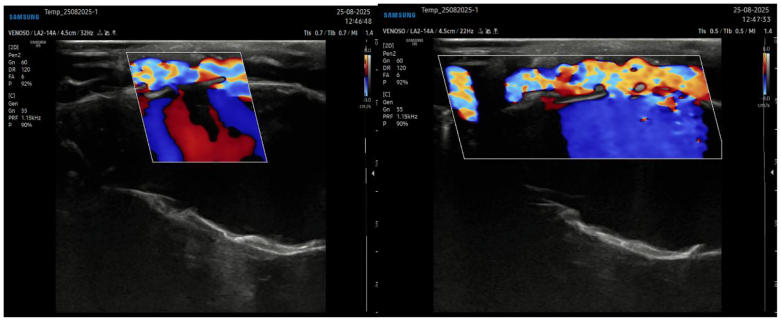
Intraoperative duplex ultrasound (DUS) examination. In the region of interest (ROI), the presence of flow in the aneurysmal sac is appreciate. The presence of flow in stent graft in the lower part is present.
